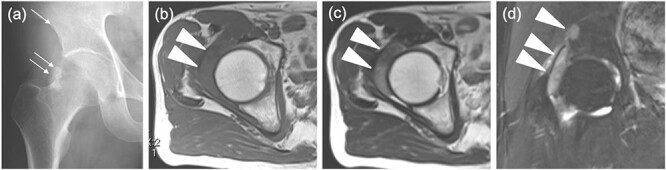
Images before the arthroscopic surgery. (a) A radiograph showed the calcification at the anterolateral side of the right femoral neck (two arrows) and a radiolucent shadow of the pelvic bone (an arrow). Axial (b) T1-, (c) T2-weighted image (T1WI, T2WI) and (d) coronal T2 fat-saturated (T2 fs) image revealed the intra-articular lesion in the right hip joint (two headless arrows). The mass was 40mm×10mm×42mm and isointense, heterogenous and hyperintense to skeletal muscle on T1WI and T2WIand T2 fs images respectively. T2WI showed triple signal intensity sign9. In addition, the coronal T2 fs image showed a pelvic bone lesion (a headless arrow).
